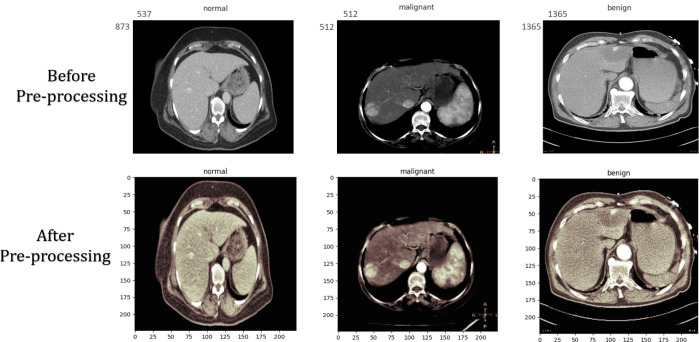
Preprocessing results for CT liver tumor images. Example CT scans before and after applying preprocessing (bilateral filtering and histogram equalization), demonstrating noise reduction and improved contrast.
